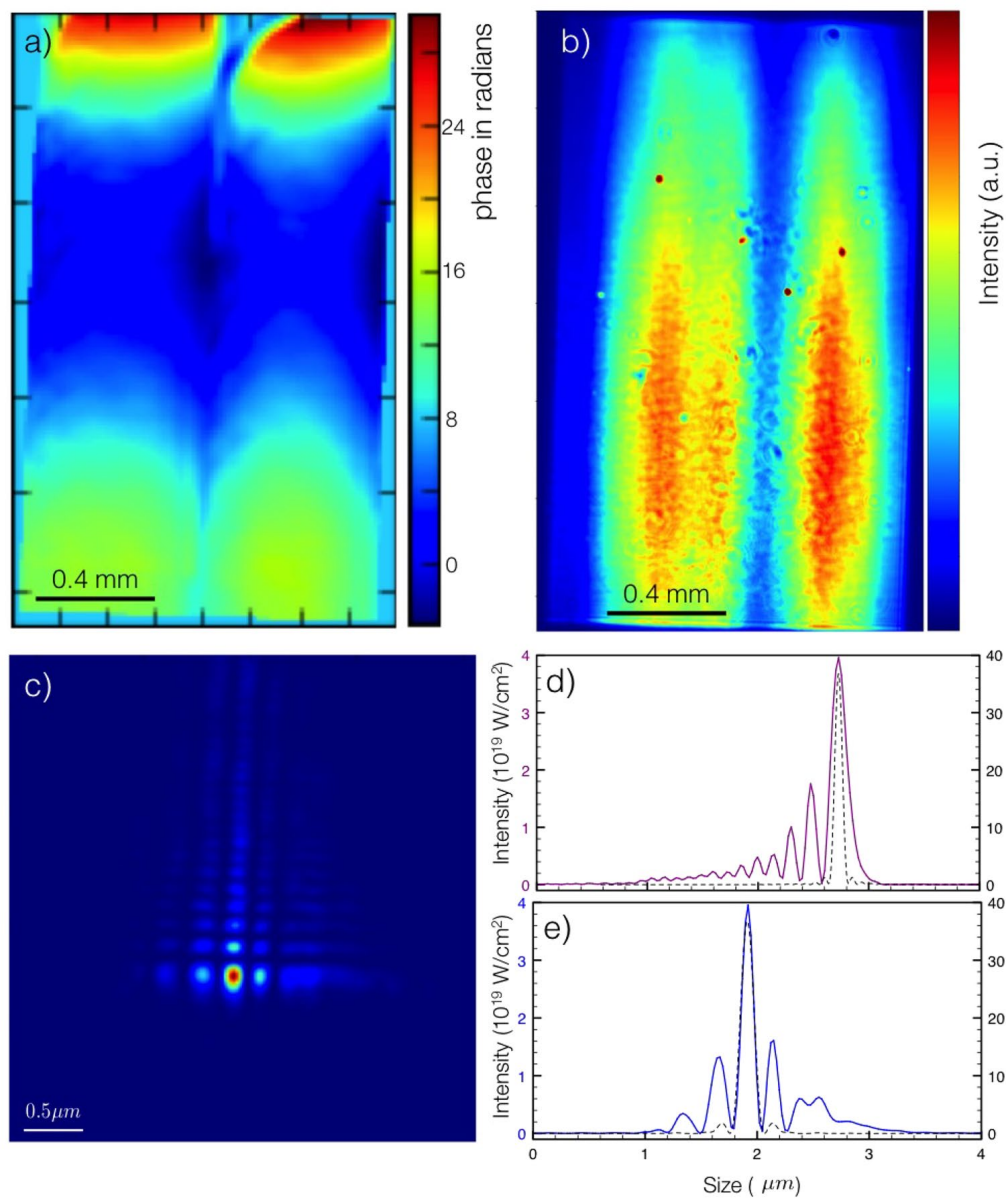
(a) Wavefront of the x-ray beam, (b) Measured intensity of the beam. (c) Intensity of the focal spot at best focus. (d) Vertical lineout (red) and (e) horizontal lineout (blue) through focus, compared with the theoretical ideal focus (dashed) when no abberations would be present. The peak intensity is 3.9 10^19^ W/cm^2^ for the 3 mJ beam energy and 60 fs pulse length that was used in the experiment.
